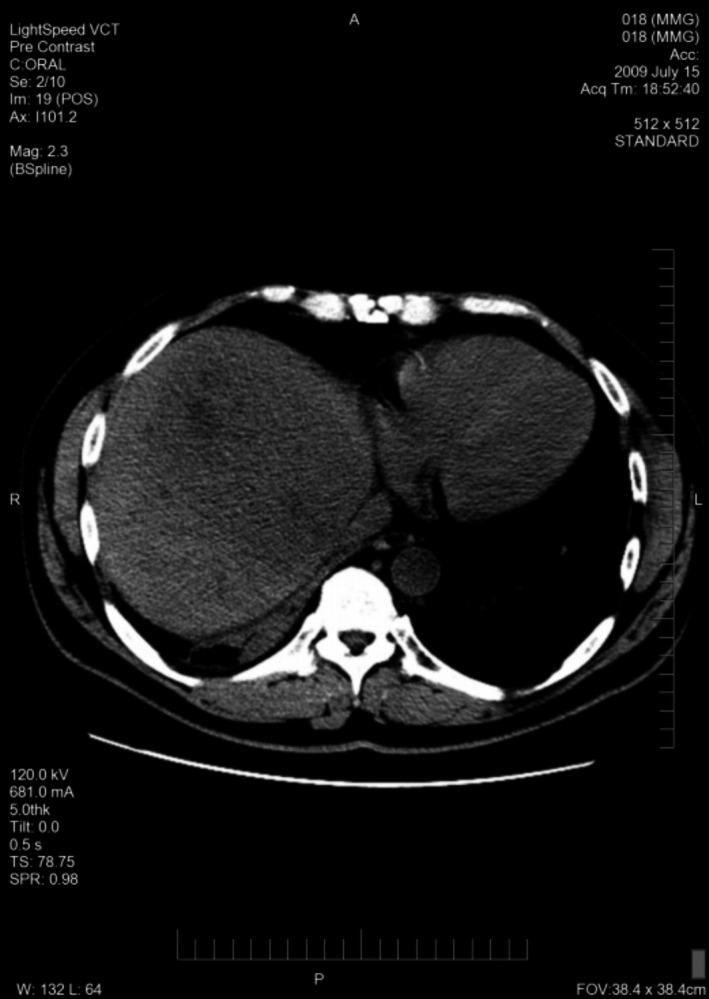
Patient 1 with Hepatocellular Carcinoma (HCC)—Baseline CT images without contrast, tumor at baseline (prior to trial enrollment) was measured at least 13.3 X 9.5 cm in segments 8 and 4a.
